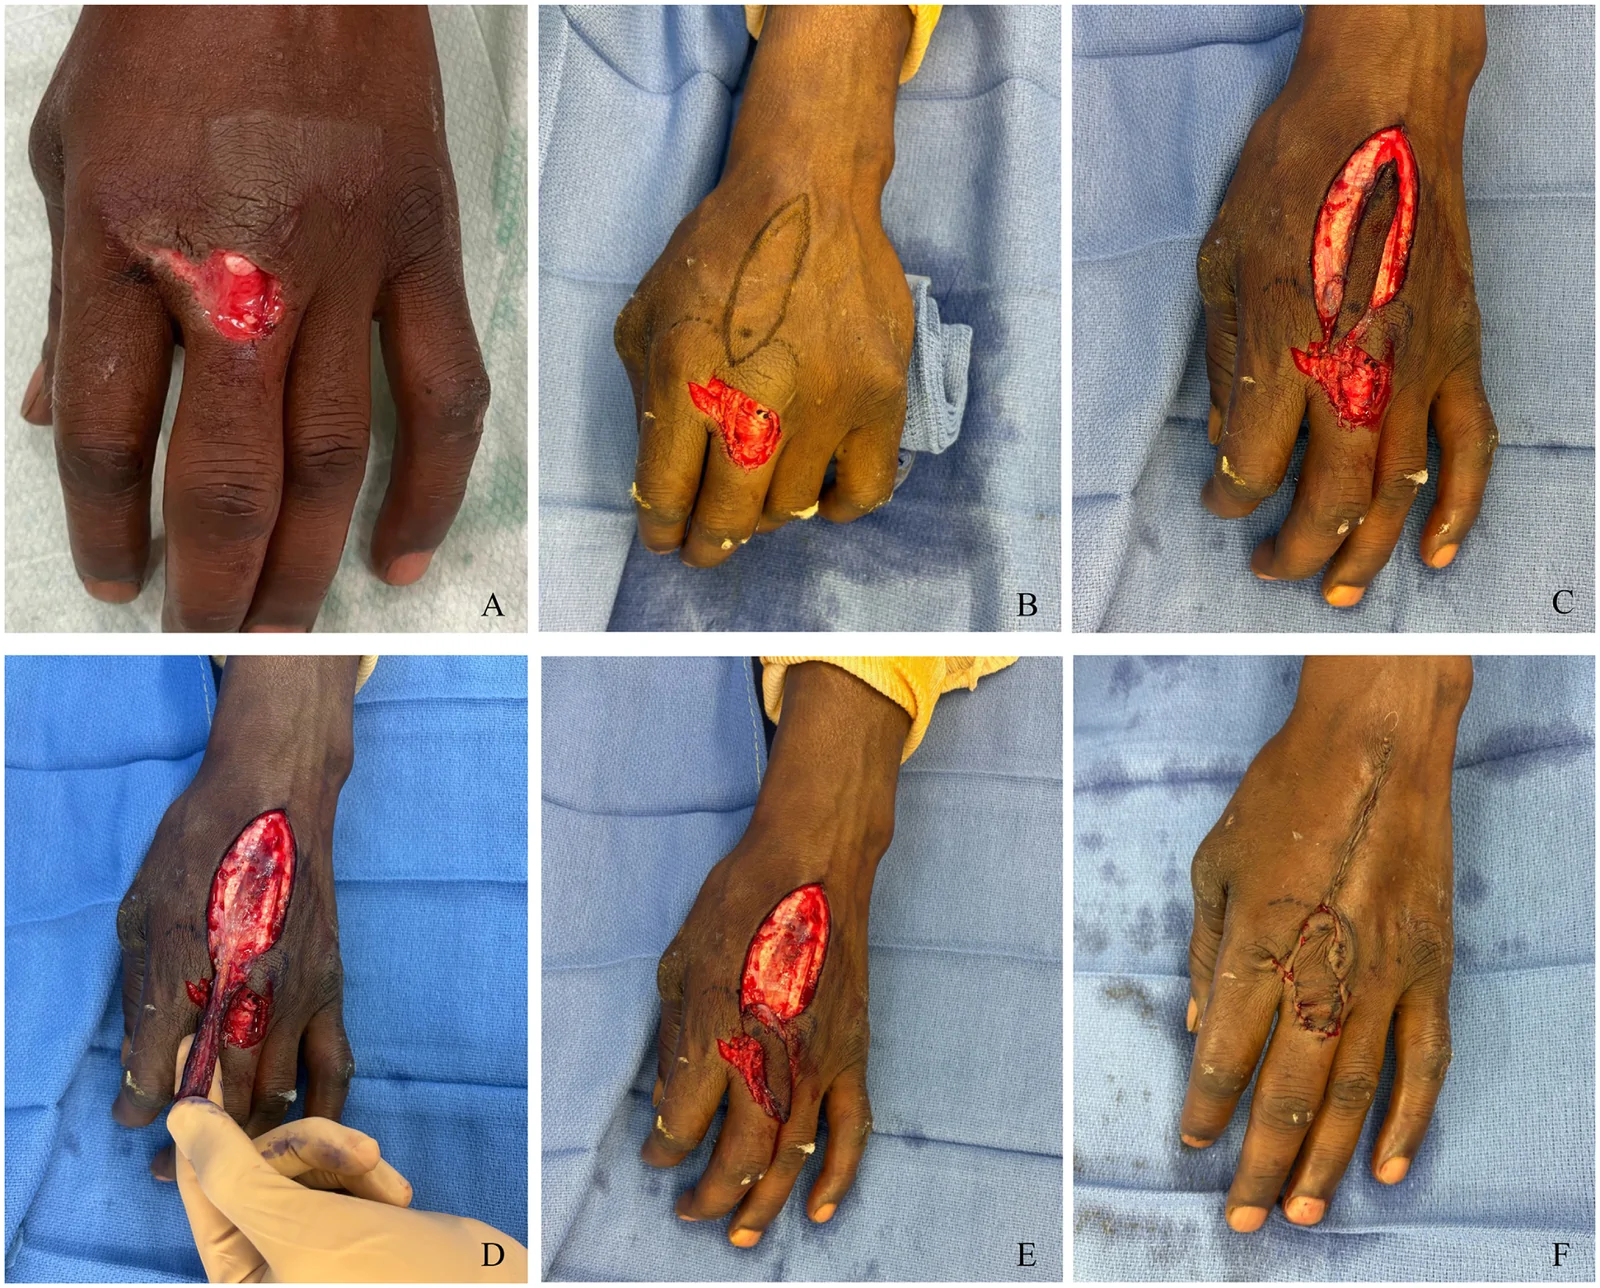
Dorsal metacarpal artery perforator (DMAP) flap for extensor tendon coverage. (A) Dorsal defect over the third finger demonstrating the complete extensor tendon rupture at the level of the metacarpophalangeal joint. (B) Extensor tendon repair using 4-0 non-absorbable U-sutures. Elliptical design of the perforator flap based on the second intermetacarpal perforator. The vascular anatomy at this level is highly constant, making preoperative Doppler mapping unnecessary. (C) Superficial skin incision along the flap design, showing continuity of the dorsal aponeurosis. (D) Intraoperative view of the flap elevated superficial to the dorsal interosseous aponeurosis and the extensor peritendinous plane, with identification of the DMCA perforator pedicle. Complete microskeletonization of the pedicle, separating the artery and vein, is unsafe in the hand; maintaining a cuff of perivascular adipose tissue is recommended to preserve pedicle integrity and reduce the risk of vasospasm or injury. (E) Intraoperative view showing preservation of the dorsal interosseous aponeurosis and peritendinous plane. Flap rotation of approximately 160° was achieved without fascial release or inclusion of excessive peripedicular fat. (F) Postoperative appearance on day 2 after inset of the DMCA perforator flap, demonstrating tension-free closure and a satisfactory dorsal contour. *Printed with permission; copyrights retained by Elise Lupon, MD, PhD.*
